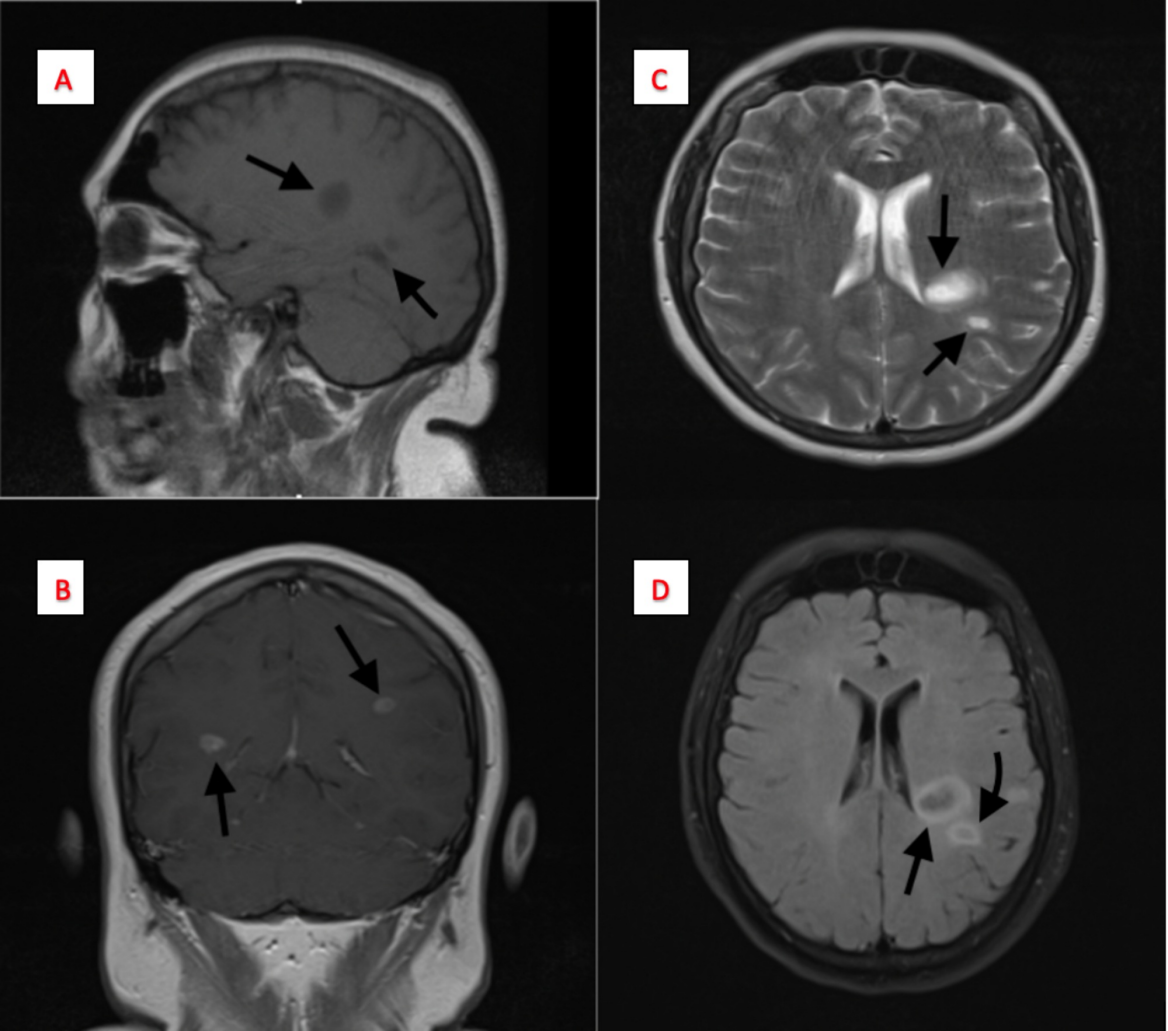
Magnetic resonance imaging (MRI) of the brain with/without contrast Panel A: Displays a sagittal T1 image with two hypodense lesions; Panel B: Displays a T1 contrast enhanced coronal image of lesions in both cerebral hemispheres; Panel C: Displays a T2-weighted image with two (16 x 22 mm and 7 x 12 mm) periatrial ring-enhancing lesions in the left periatrial white matter; Panel D: Displays flair image of the periventricular lesion.
